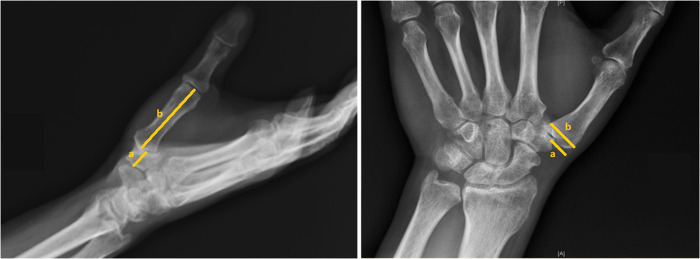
Radiograph of CMC OA with loss of joint space and radial dislocation. (Left) (a): axis of the trapezium and (b): axis of the first metacarpal; (right) (a): uncovered length of trapezium and (b): total length of trapezium.
